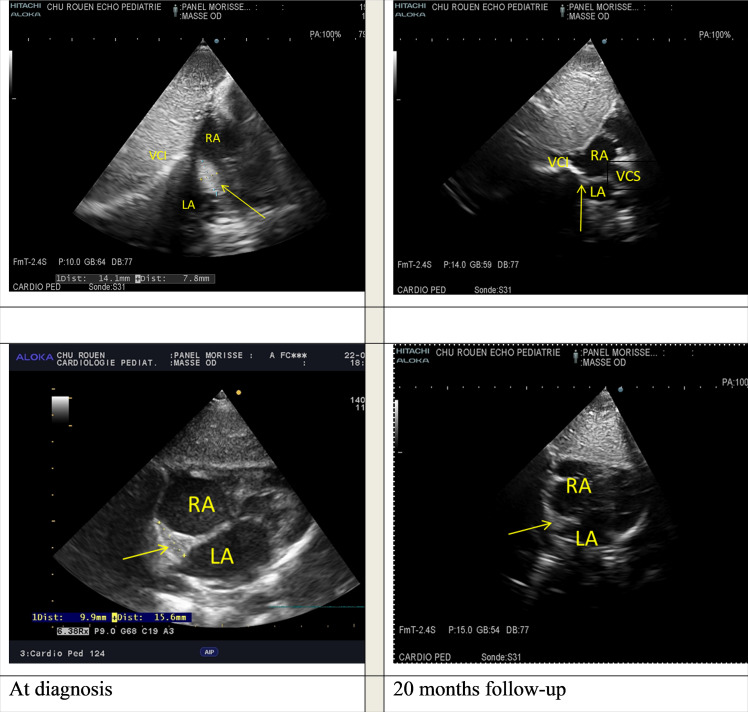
Echocardiographic views comparison of intracardial mass (yellow arrow) between the diagnosis and 20-months follow-up. RA, Right Atrium; LA, Left Atrium; IVC, Inferior Vena Cava; SVC, Superior Vena Cava.
